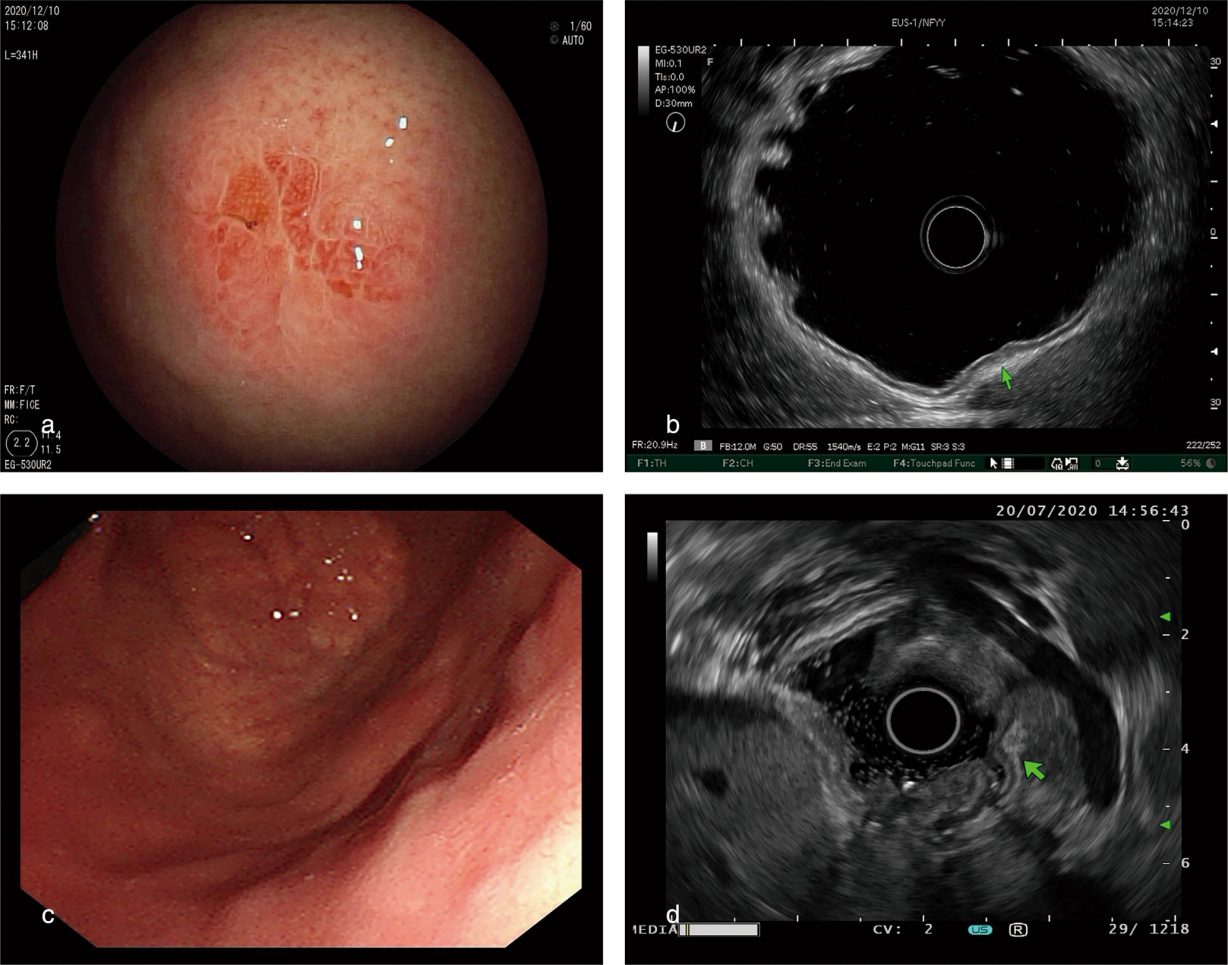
Representative images of white‐light imaging (WLI) and endoscopic ultrasound (EUS) findings. WLI showed SMT‐like type lesions (a, c). EUS showed lesions located in the deep layer of gastric mucosa or gastric submucosa, with hypoecho (b, d).
